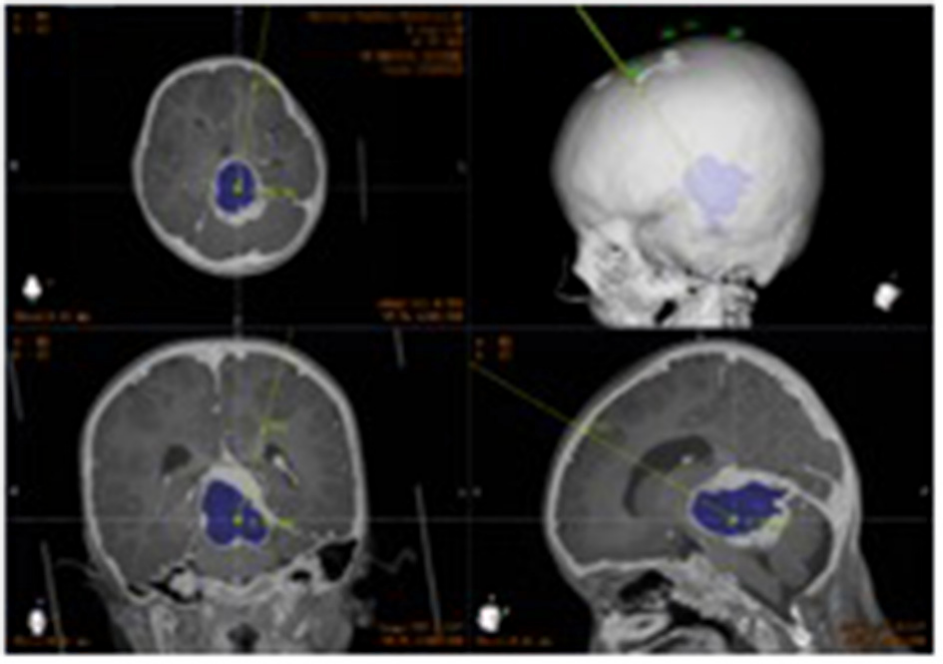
Postoperative CT. Postoperative image fusion, 3D reconstruction of the position of the intracranial drainage tube.
